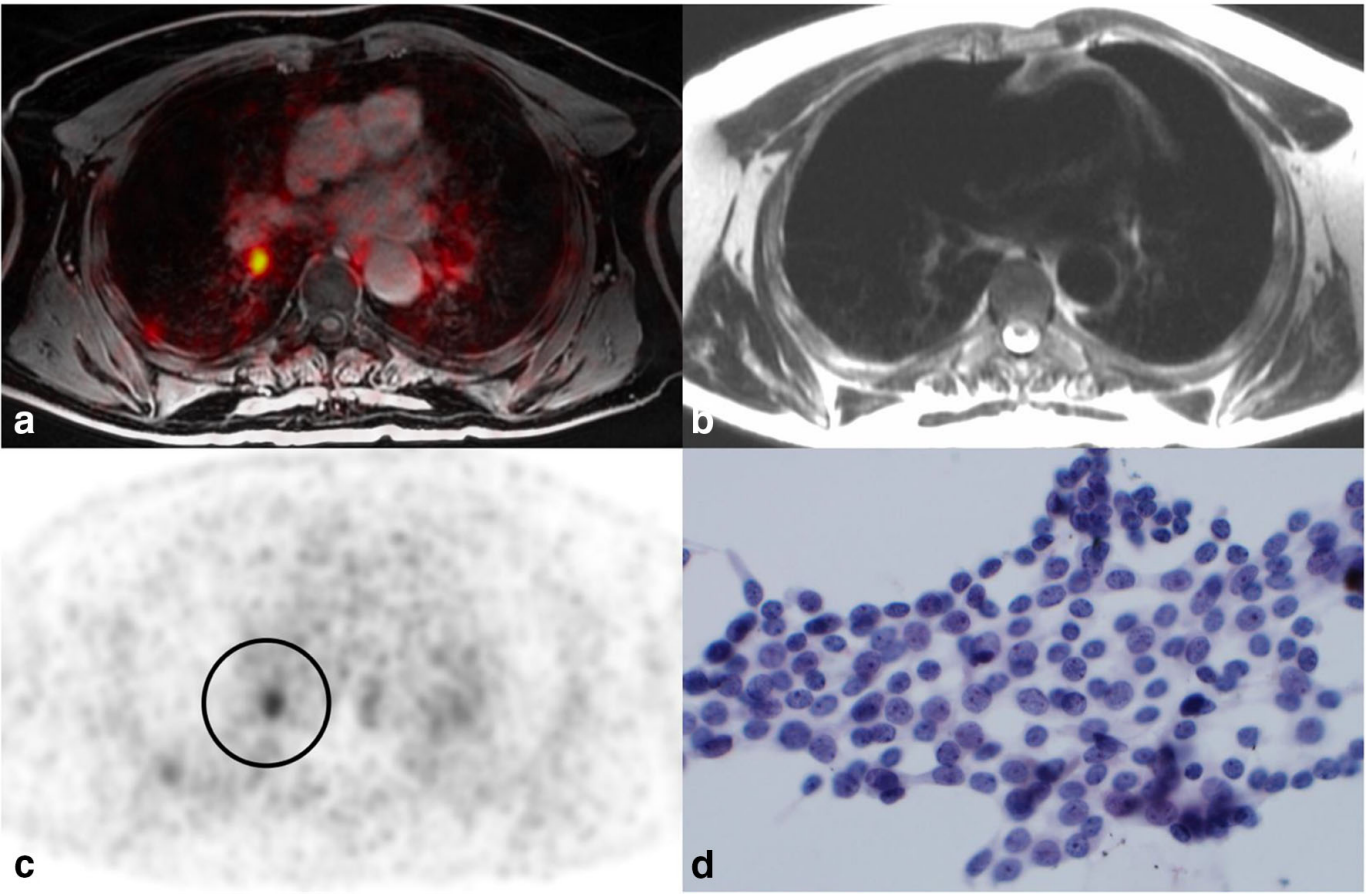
68-year-old man post-radiotherapy therapy with focal uptake (SUV_max_ 13.0) noted in the right hilum on [^68^Ga]Ga-PSMA-11 PET (a and c, circle) (PSA 4.7 ng/mL, MRI FP 8). Single shot fast spin echo (b) does not visualize the lesion highlighting limitations of PET/MRI for characterization of small lung findings. Transbronchial biopsy demonstrates cuboidal epithelium (d) with adjacent prominent glands typical for a bronchogenic cyst.
